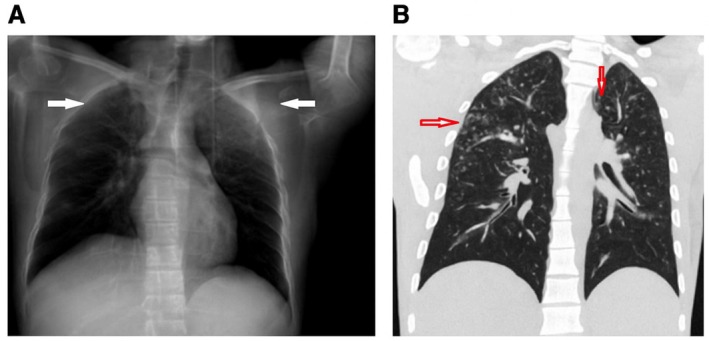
(A) Chest X‐ray showing bilateral apical opacities (white arrows). (B) Coronal High‐Resolution Computed Tomography (HRCT) of the thorax demonstrating micronodular ground‐glass opacities in the bilateral upper and lower lobes, more prominent in the posterior regions (red arrows).
